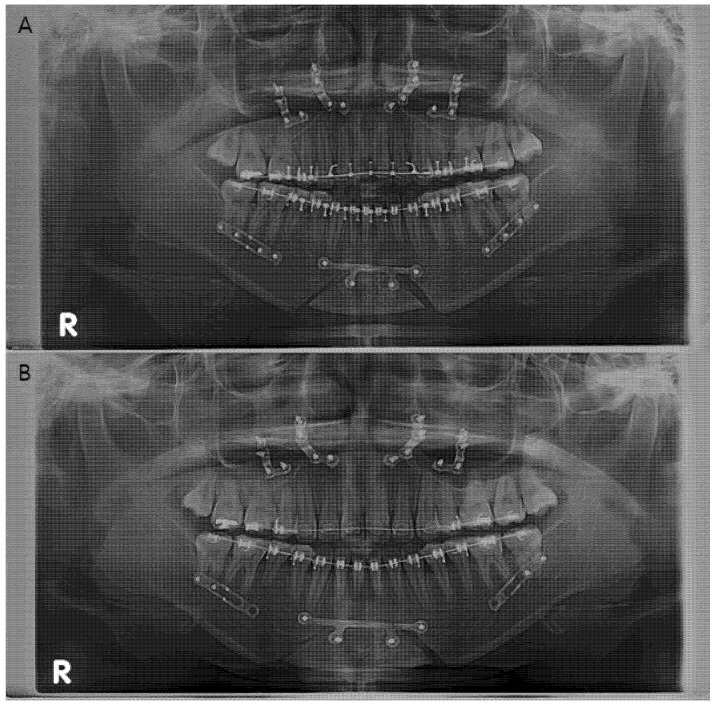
(A) Panoramic radiograph of a patient (Patient no. 3) who had undergone orthognathic surgery. (B) Panoramic radiograph after early screw removal in the distal segment. “R” on the panoramic radiograph means the right side of the patient.
